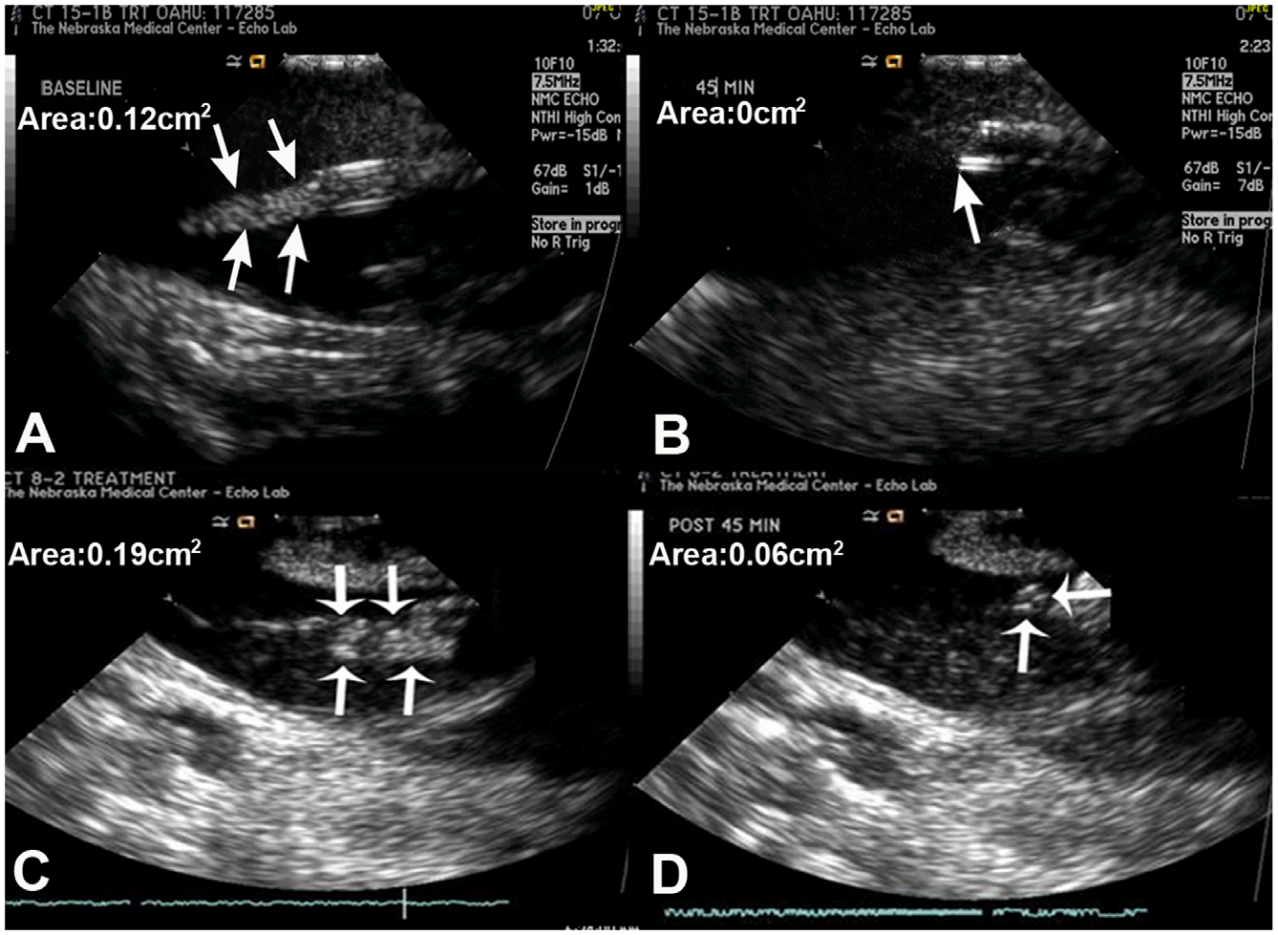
Intracardiac echocardiography before and after treatment. Examples of intracardiac echocardiographic images of the CVC tip before (panels A, C) and after (panels B, D) long pulse ultrasound and microbubble treatments demonstrating varying grades of thrombus reduction after treatment. Thrombus dissolution was complete (Panel B), near complete (Panel D).
